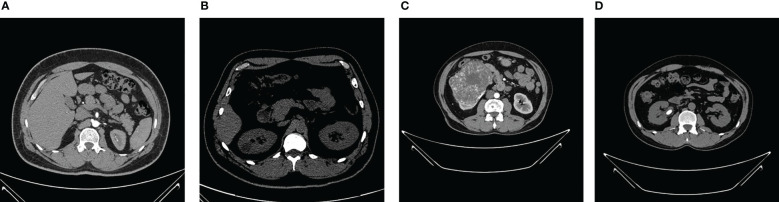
CT Kidney dataset (A) Normal (B) Cyst (C) Tumor (D) Stone.
